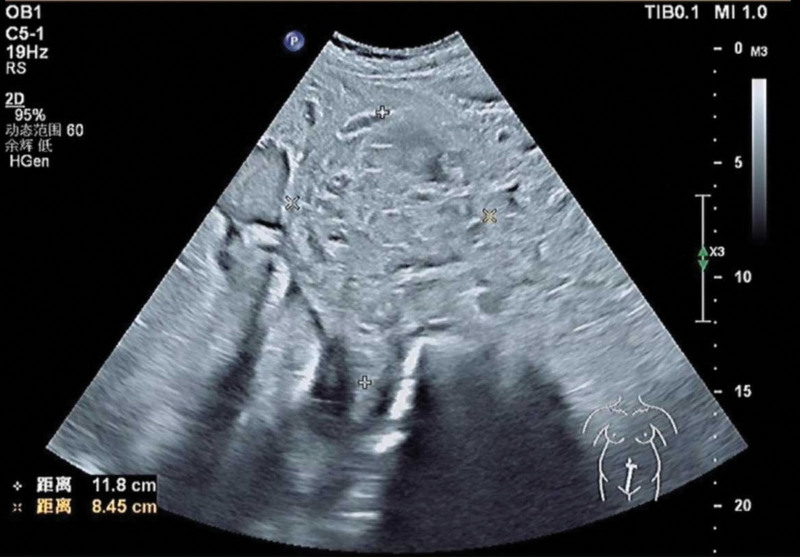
Obstetric ultrasound images about uterine leiomyoma.
